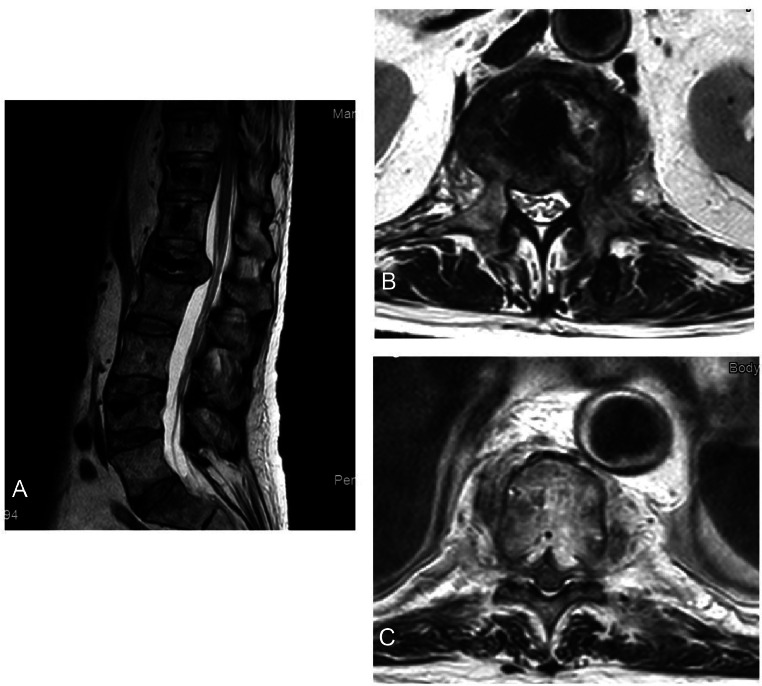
Sagittal (A) and axial (B and C) contrast enhanced MRI of the thoracolumbar spine 6 weeks after surgeries showing infiltrative osseous lesions involving L1 and T10 vertebral body with soft tissue component compression the cord at L1 (B) and at T10 (C).
